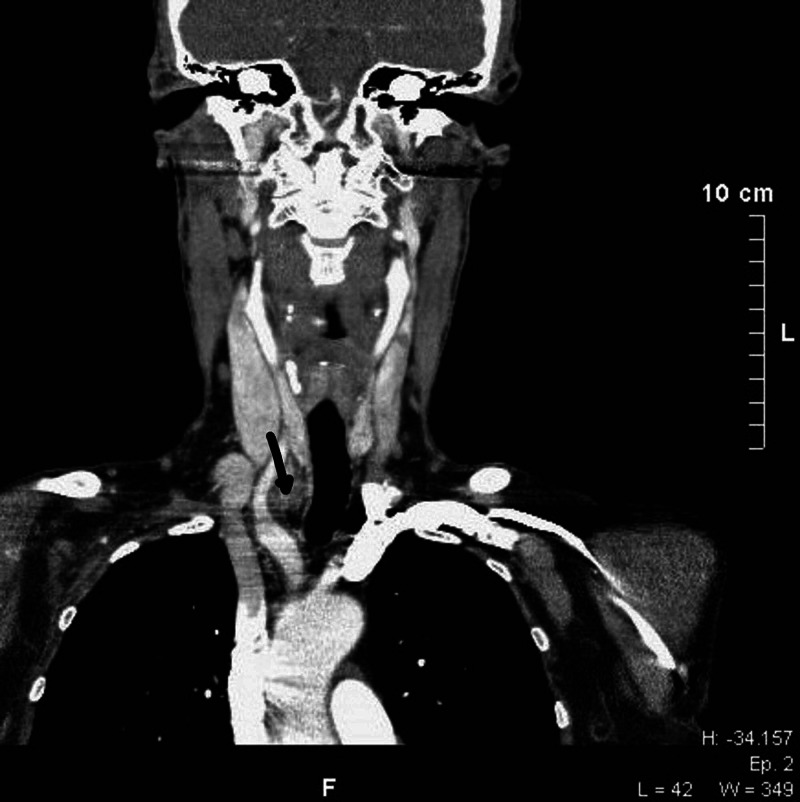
Three-dimensional CT of the tumor The arrow indicates the position in close proximity with the innominate artery CT: computed tomography
